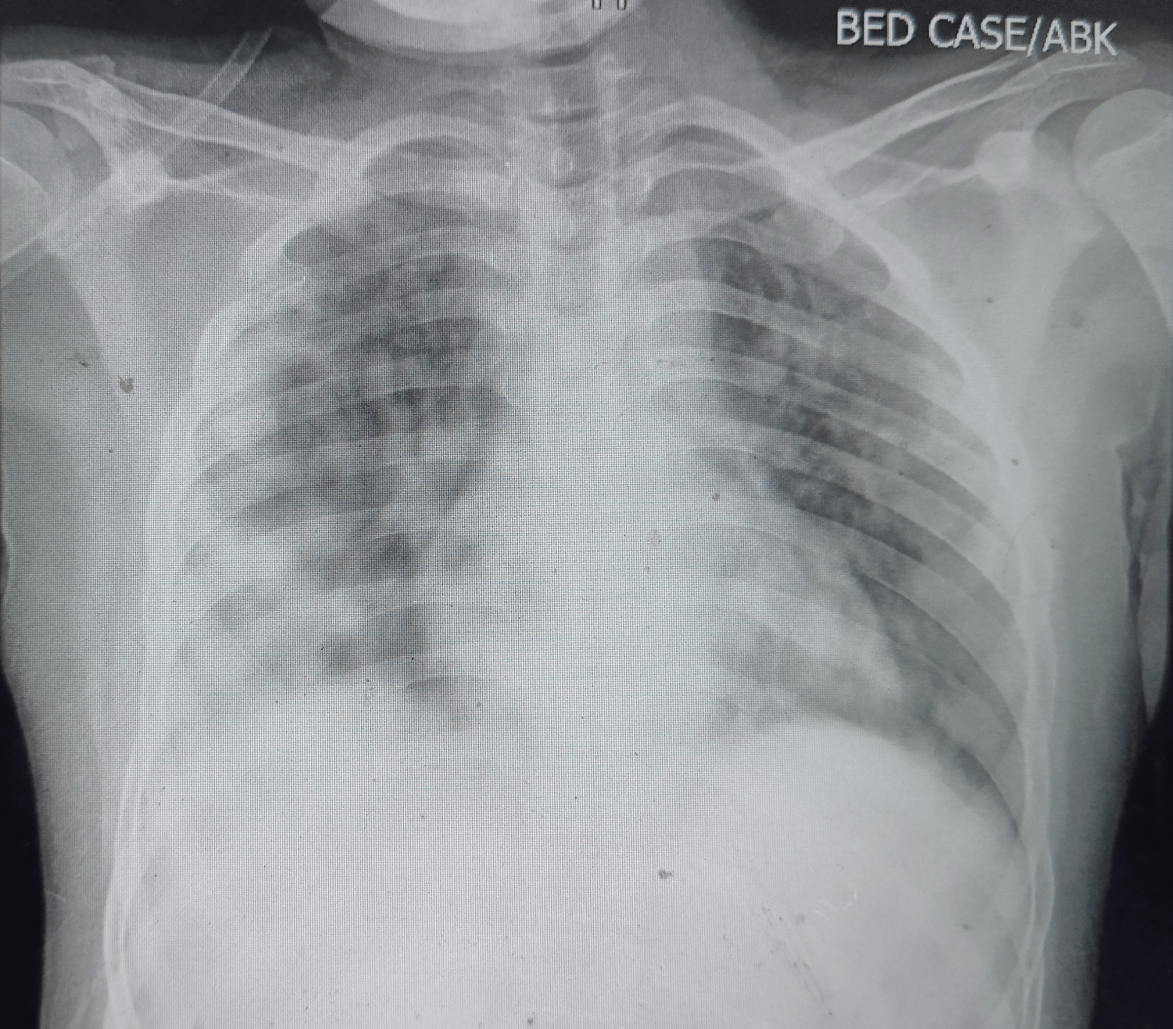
Chest X-ray on day 3 of hospitalization
